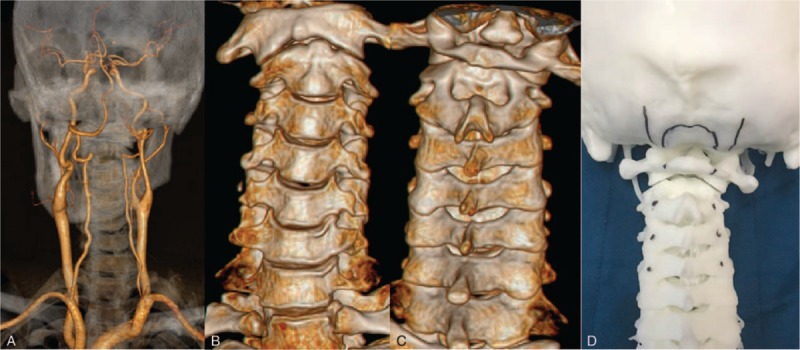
(A) Preoperative cervical CTA to evaluate the relationship between the important blood vessels and bone in the cervical area. (B and C) 3D CT of the cervical was performed to evaluate the bony structure. (D) A 3D printing model of the patient's occipitocervical area was constructed using CTA and 3D-CT. 3D = 3-dimensional, CTA = computed tomography angiography.
